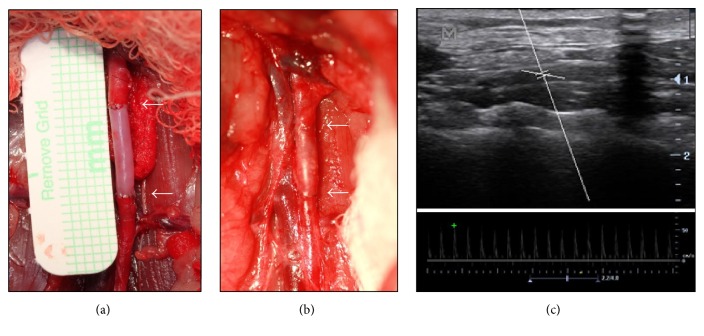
Implantation and* in vivo *imaging of the graft. Picture of the same scaffold immediately after implantation (a) and 6 months later (b), showing integration of the graft. The white arrows point to the anastomotic sites. (c)* In vivo* ultrasound before sacrifice 6 months after implantation confirming aortic blood flow. The two parallel lines in the central aspect of the aorta are the region in which the velocity is measured (Doppler gate), the long white line perpendicular to the Doppler gate is for a rapid visual identification of the Doppler gate, and the smaller white line is the Doppler angle.
